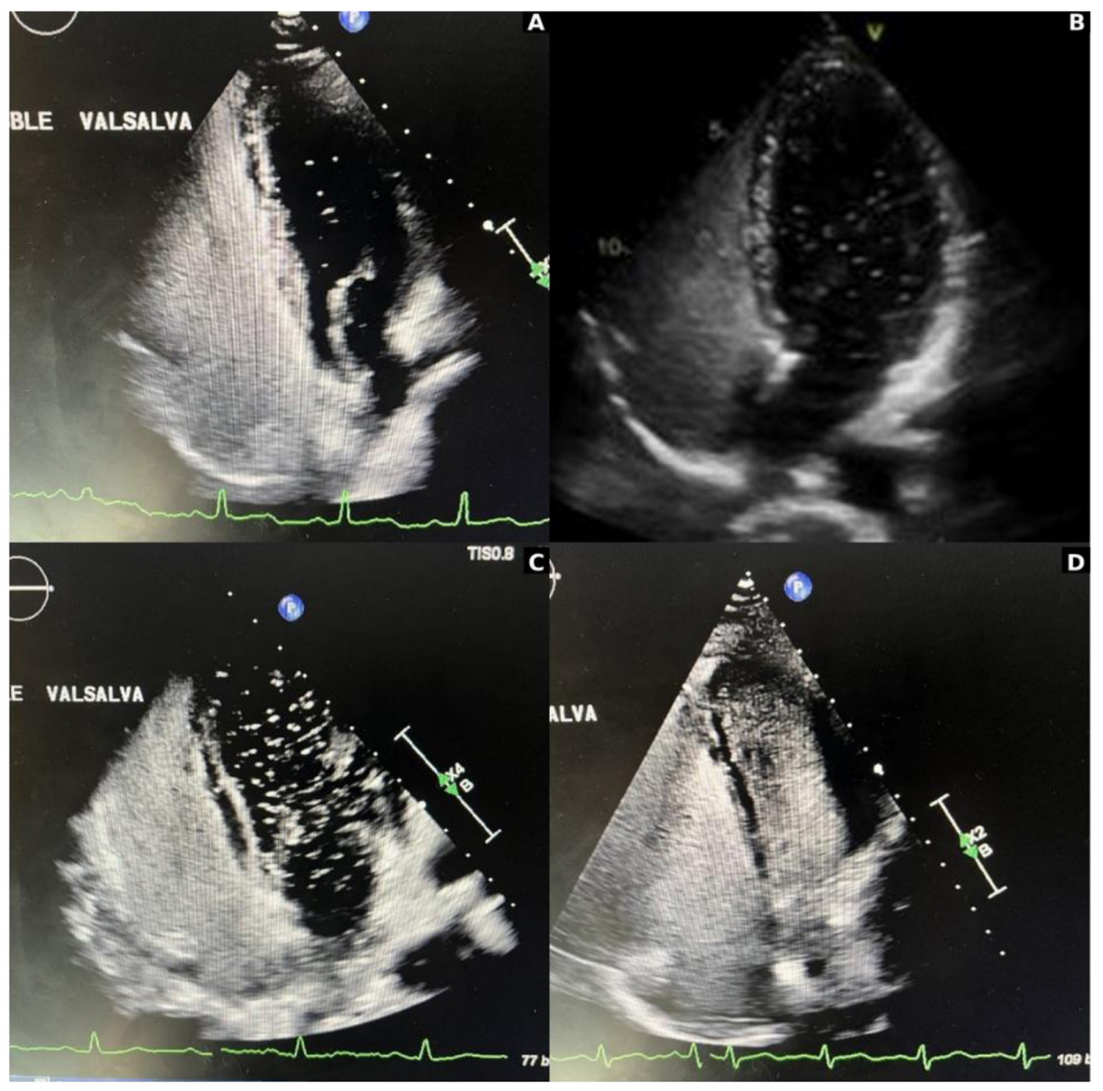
Grading of patent foramen ovale (PFO) on bubble echocardiogram: (A) small or grade 1 = 1–5 bubbles; (B) moderate or grade 2 = 6–19 bubbles; (C) large or grade 3 = ≥20 bubbles; (D) very large or grade 4 = near opacification.
